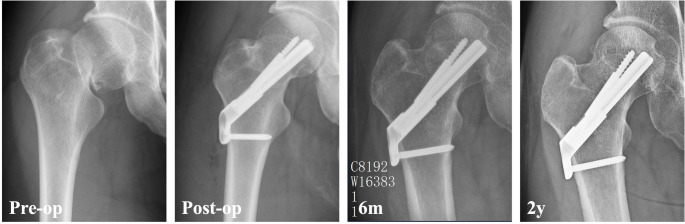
A 33-year-old male who sustained a Pauwels III femoral neck fracture was treated with femoral neck system. The radiograph taken 6 months and 2 years postoperatively revealed fracture union without complications
